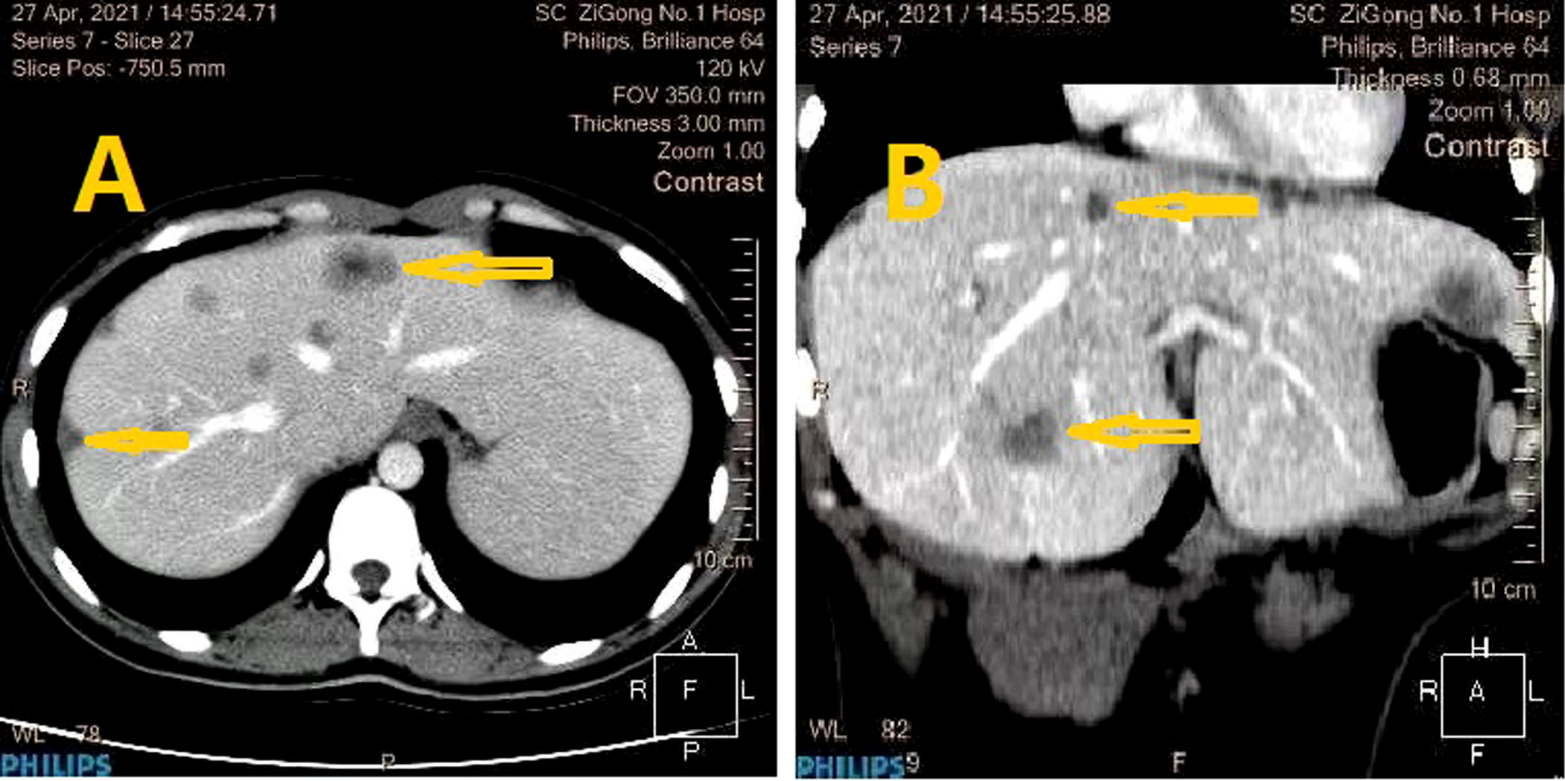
CT imaging: The metastatic liver lesion and low-density lesions in the liver was shown by CT (lesion was marked with yellow arrow) [(A), (B) reflect different anatomical layers].
